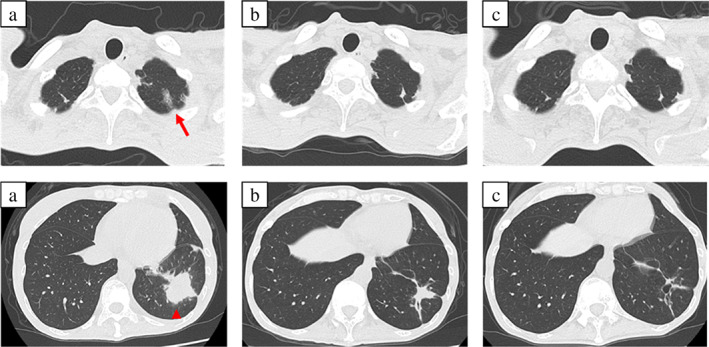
Chest computed tomography (CT) scans taken on (a) day 115, (b) day 165, and (c) day 193. Ground‐glass opacity (arrow) became organized and the nodule (arrowhead) disappeared during treatment with osimertinib.
